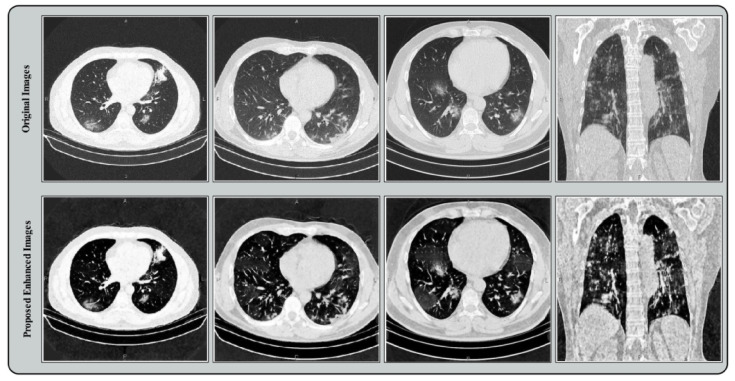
Proposed contrast enhancement effects on the original chest CT images.
